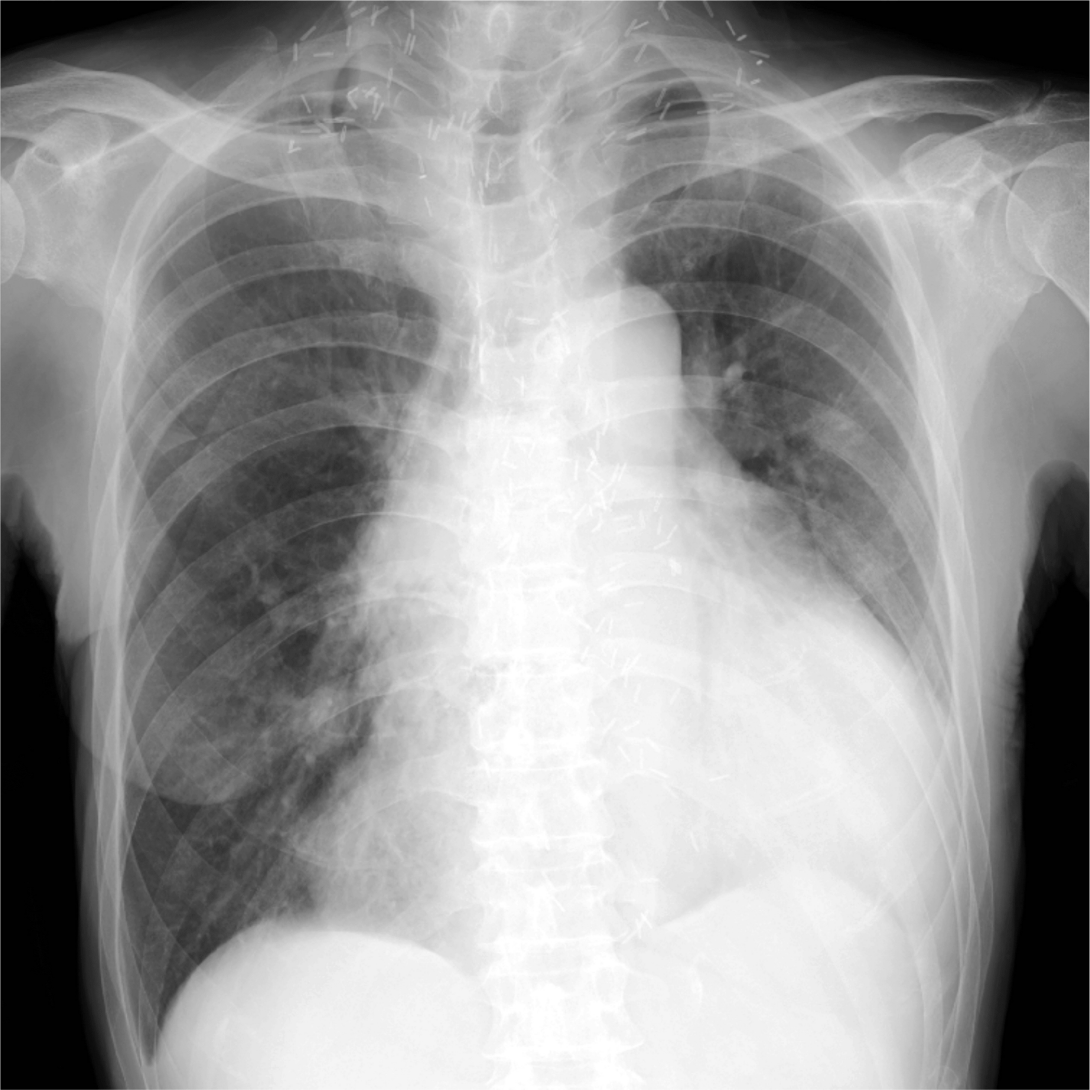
Chest radiography showing cardiac and pulmonary features. The chest radiograph highlights a cardiothoracic ratio of 69%, indicating cardiac enlargement. Both costophrenic angles appear dull, suggesting pleural effusion, consistent with the patient’s symptoms of congestive heart failure.
